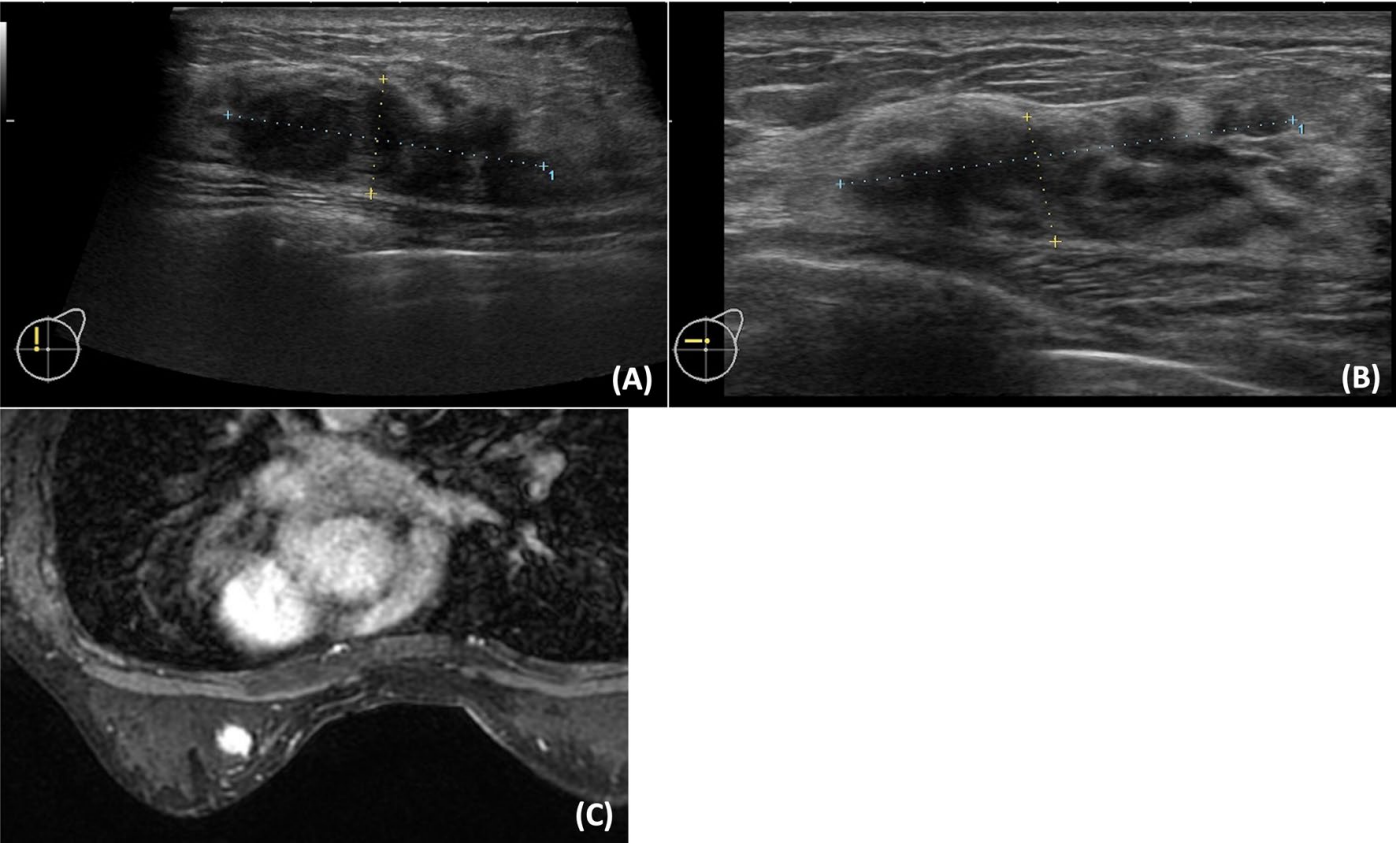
Breast ultrasonography and MRI. A A breast ultrasonography showing an irregularly shaped 36-mm low echoic mass with abundant vascular signal in the upper left medial region. B Breast ultrasound showing no increase in the mass one year and eight months after the diagnosis of IgG4-RM. C Breast MRI showing an irregularly shaped contrast-enhanced mass
